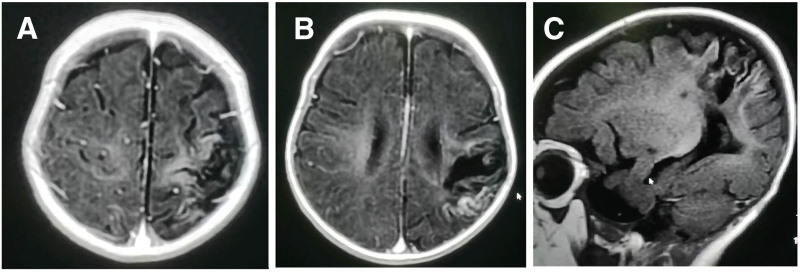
Cranial MRI: There is a loss of the left frontal parietal lobe, insula, and the left central light gray matter, with irregular morphology of the local gyri. Irregular long T1 and long T2 signals are observed, with relatively clear boundaries, measuring approximately 31mm42mm26mm.
